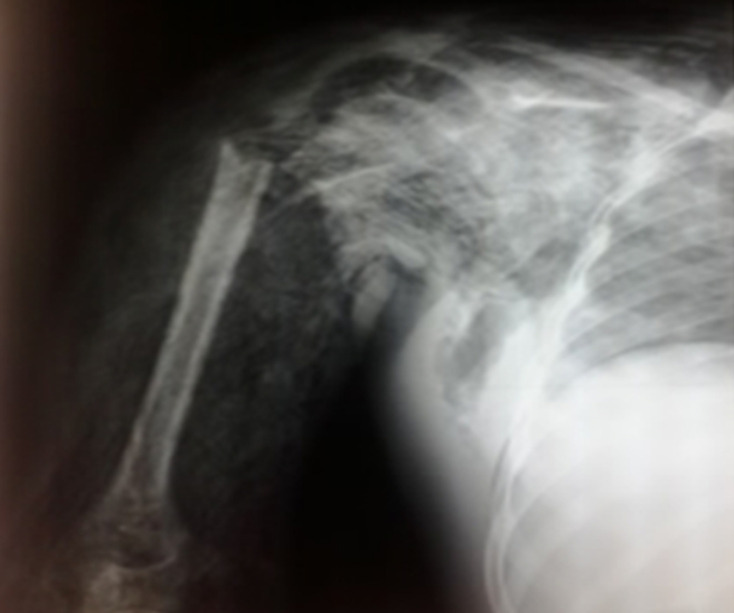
image radiographique pré-opératoire de la gangrène mixte du bras droit étendue à l'épaule après une fracture du col chirurgical de l'humérus
